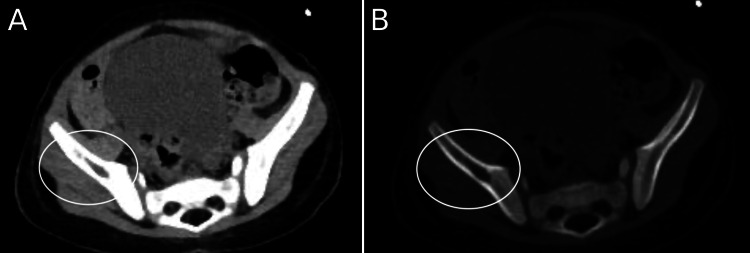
CT scan showing 1 cm lytic lesion in the right iliac wing.
